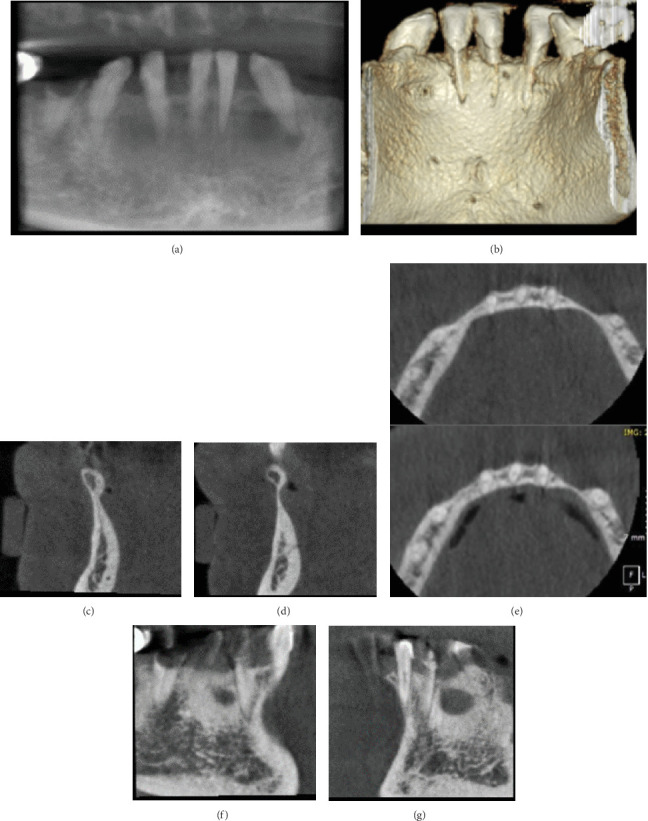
Case 3. CBCT scan. (a) Panoramic reconstruction of CBCT showing bilateral bone radiolucency in the anterior mandible. (b) 3D rendering. MPR views: (c, d) corrected coronal, (f, g) corrected sagittal, and (e) two levels of axial sections. These images show bilateral lingual bone depression for area between Teeth #32 and #33 and area between Teeth #42 and #43. These depressions bilaterally representing the differential diagnosis of Stafne bone defect.
